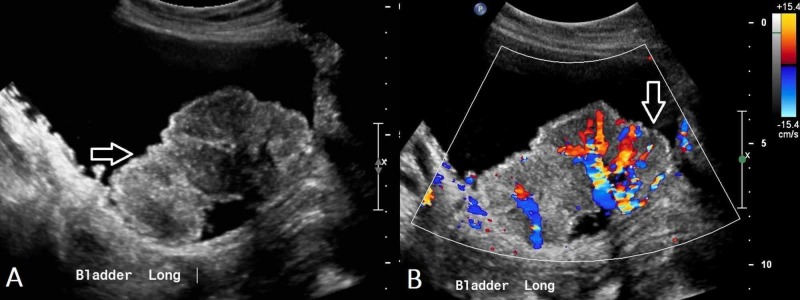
Transitional cell carcinoma of the bladder (US) (A) Ultrasound pelvis demonstrates an irregular, heterogenous within the urinary bladder with multiple smaller polypoidal lesions seen adjacent which is suggestive of urinary bladder cancer. (B) Vascularity is demonstrated within this mass.
